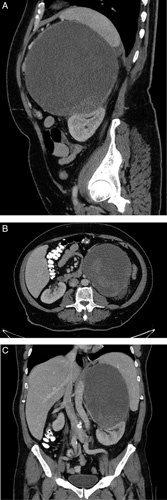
Sagittal, axial, and coronal computed tomography (CT) images showing heterogenous left apical mass. Sagittal (A), axial (B), and coronal (C) CT images show a solid tumor measuring 17 cm in diameter at the upper pole of the left kidney, with peripheral calcifications. It was found to be abutting the spleen and displacing the left kidney inferiorly, as well as the tail of the pancreas anteriorly.
